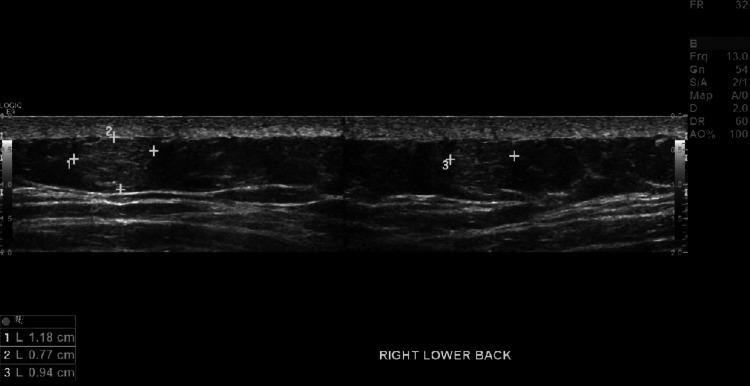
Ultrasound image of a 1.18 x 0.94 x 0.77 cm right lower back soft tissue mass
